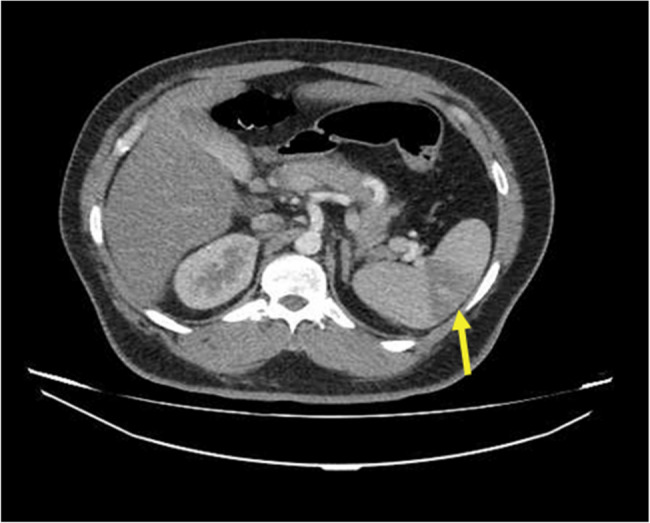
Axial Abdominopelvic CT with intravenous contrast. Splenic infarction visible as a wedge-shaped hypodense area in the spleen. (Arrow indicates area of infarction)
